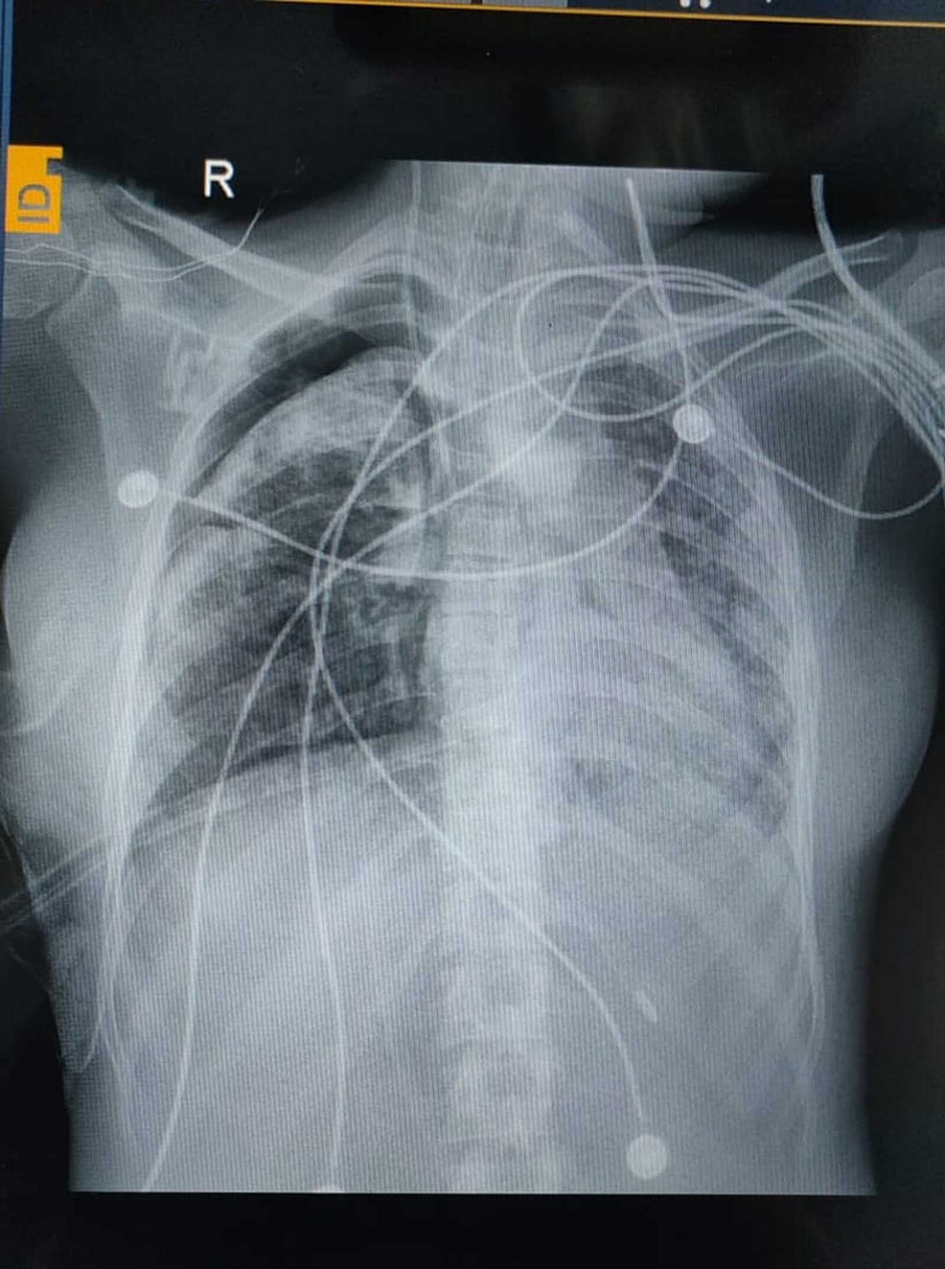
Chest radiograph on day five of admission Chest radiograph shows right-sided pneumothorax.
